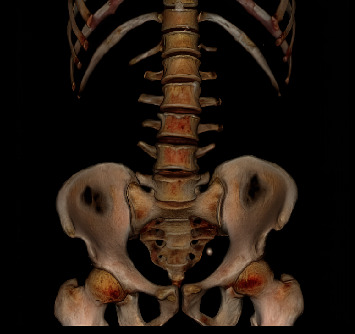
Three-dimensional CT reconstruction shows an 18-mm left lower ureteric stone.
